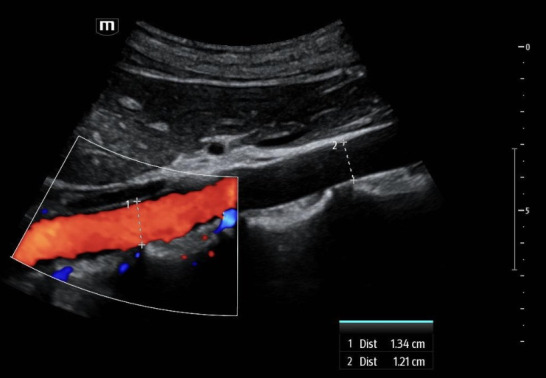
Point of care ultrasound (POCUS) images with color Doppler of a 22-year-old female standardized patient showing a sagittal view of the abdominal aorta. Color flow clarifies which of two neighboring linear hyperechoic structures is the true wall of the abdominal aorta.
